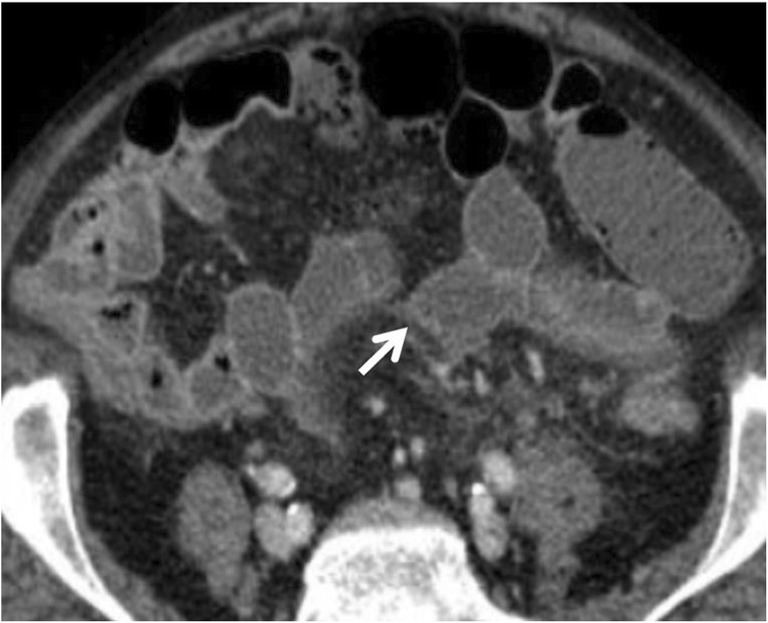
Bowel wall enhancement. Contrast-enhanced axial CT scan shows normal dilated loops with a diffuse hypointense segment of small bowel wall (white arrow) with reduction of bowel wall thickness due to superior mesenteric artery occlusion
